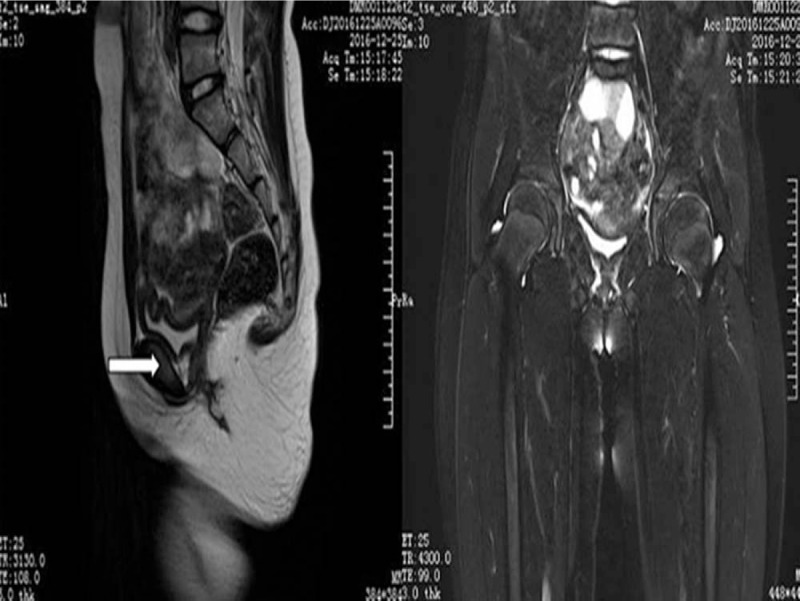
T2-weighted contrast-enhanced magnetic resonance imaging (MRI) results: preoperative (axial): a cystic lesion with a solid component was detected in the uterine cervix (arrow). An estimated measurement of 11mm × 16mm × 13 mm solid occupation can be seen at the cervix. The tumor mass was arising from the cervix into the vagina without breaking through the serous layer. preoperative (coronal): a highly suspicious solid malignant lesion occupying the tip of the cervix. MRI = magnetic resonance imaging.
